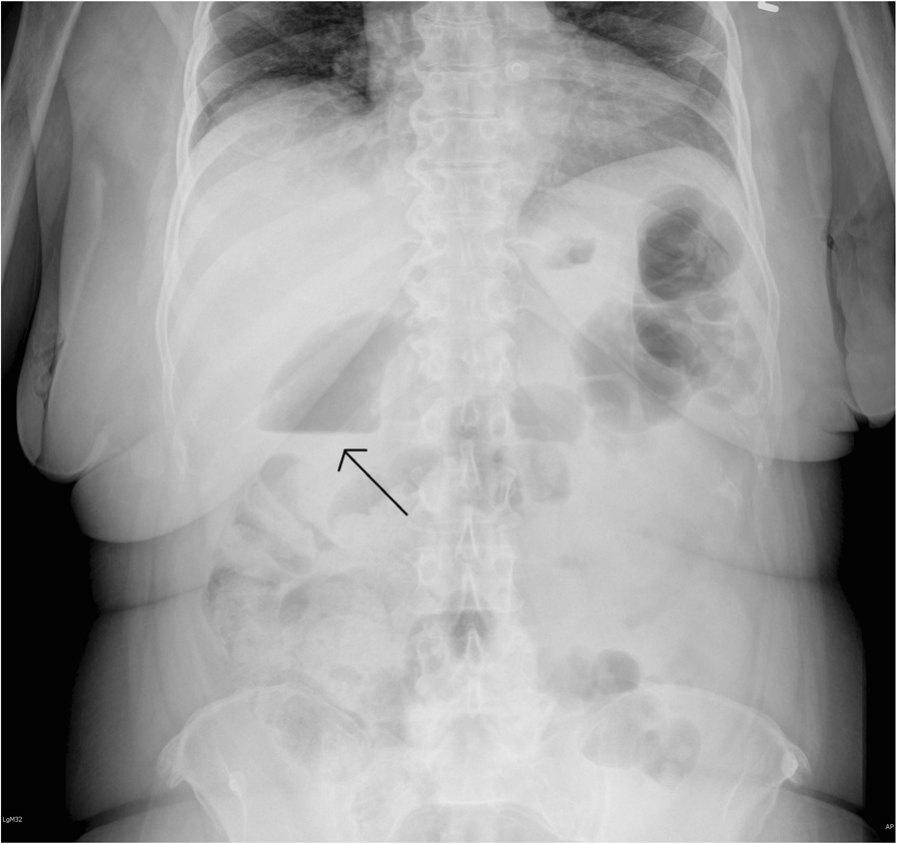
Upright abdominal radiograph demonstrating an air-fluid level in the gallbladder, diagnostic for emphysematous cholecystitis.
